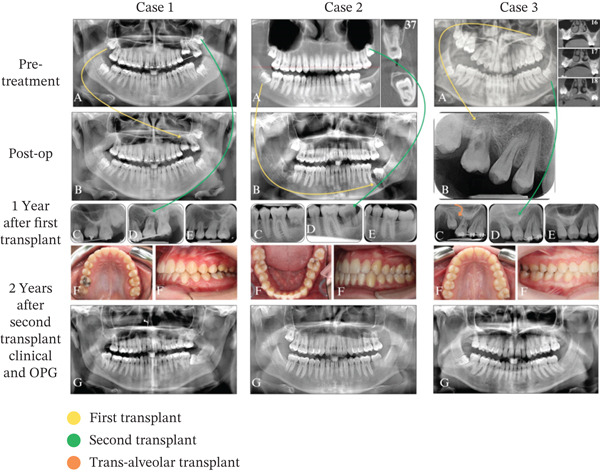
Initial presentation and treatment progression of three patients undergoing two‐stage autotransplantation with 3D planning and orthodontic coordination. (A) Initial panoramic and CBCT radiographs showing root development, recipient site condition, and donor tooth position before treatment. (B) Immediate postoperative radiographs after the first autotransplantation. (C) One‐year follow‐up radiographs after the first autotransplantation. (D) Immediate postoperative radiographs after the second autotransplantation. (E) Two‐year follow‐up radiographs after the second autotransplantation. (F) Intraoral photographs (occlusal and buccal views) demonstrating arch form and esthetic outcome. (G) Final panoramic radiographs at 2 years after the second autotransplantation follow‐up. Colored arrows indicate the sequence and type of autotransplantation procedures: Yellow denotes the first autotransplantation, green denotes the second autotransplantation, and orange denotes transalveolar autotransplantation.
